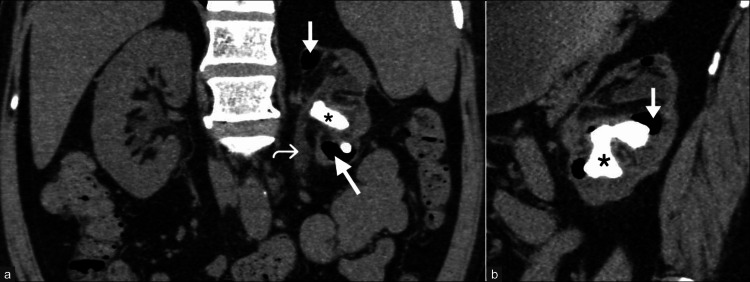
Emphysematous pyelonephritis with staghorn calculus and urinary obstruction. A 52-year-old male patient presenting with complaints of left flank pain and a thin stream of urine was diagnosed with emphysematous pyelonephritis. (a) Coronal reformatted image of non-contrast CT of the abdomen shows a relatively small left kidney with multiple pockets of air (arrows) in the renal parenchyma and pelvicalyceal system, a hyperdense calculus (asterisk) in the pelvicalyceal system, hydronephrosis, dilated ureter (curved arrow), and perinephric fat stranding. (b) Sagittal reformatted CT image demonstrates the calculus to be a "staghorn" calculus with adjacent emphysematous changes (arrow). These images are from the hospital and have not been published elsewhere. CT: computed tomography
